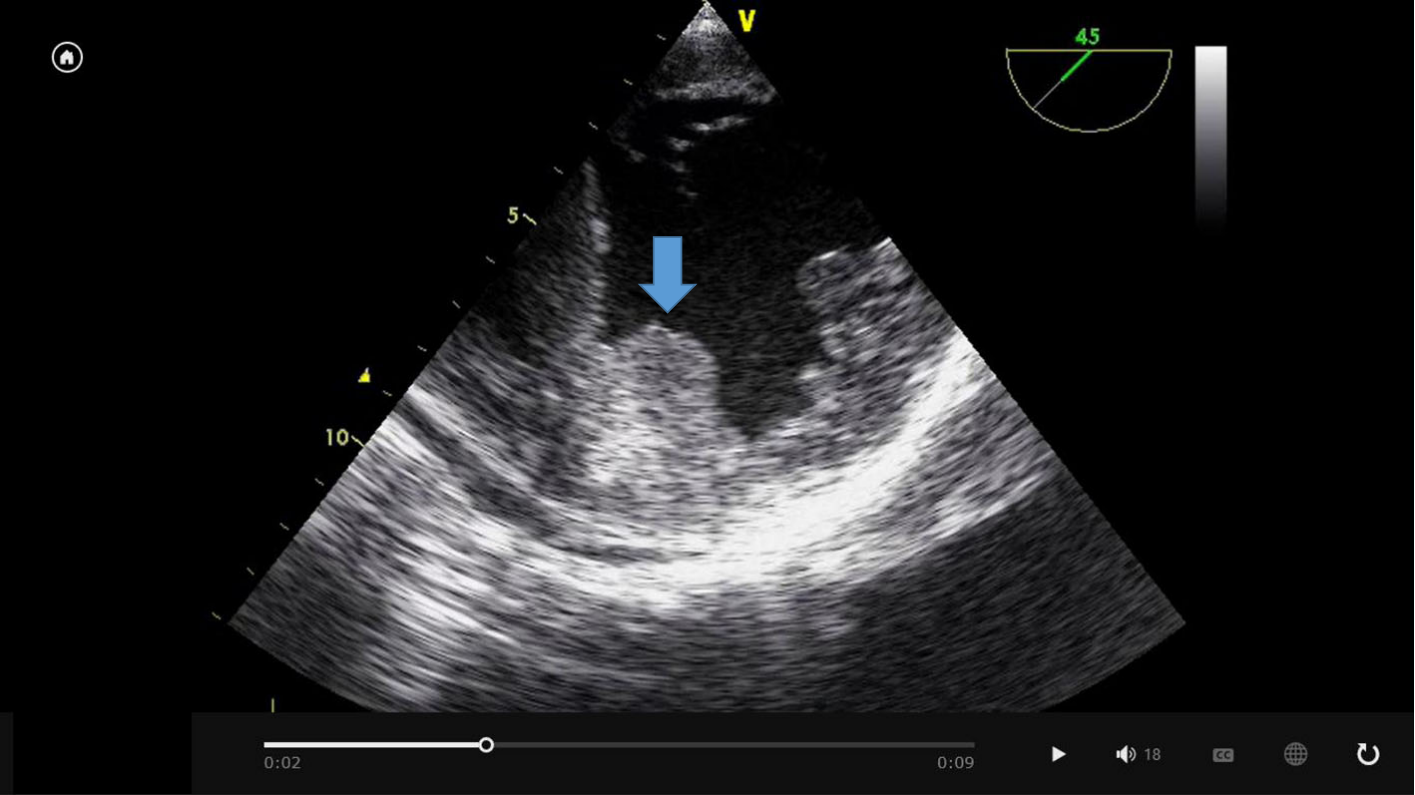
Left ventricular thrombus observed by transesophageal echocardiography under general anesthesia. The thrombus () was mobile and was located in the septal areas
